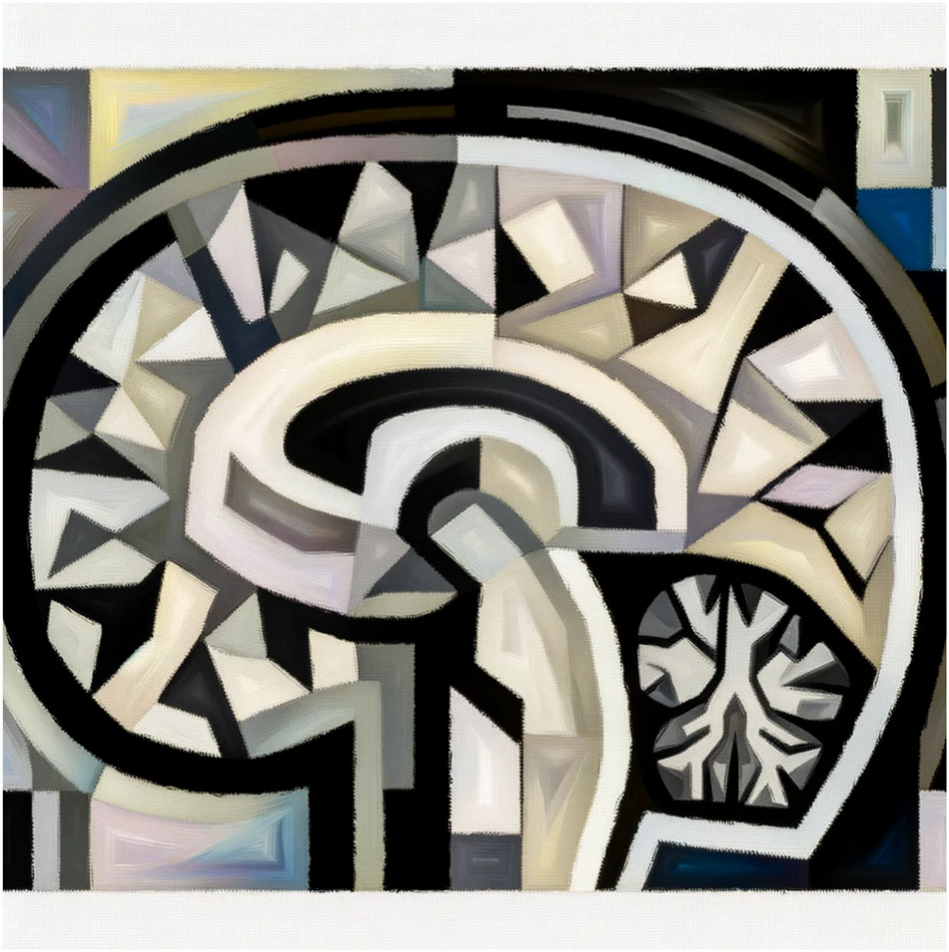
A Picasso-style representation created by OpenAI ChatGPT-4 with the prompt “create a Cubism-like magnetic resonance image of the brain”, showing the potentials of generative AI even in the creation of art pictures
